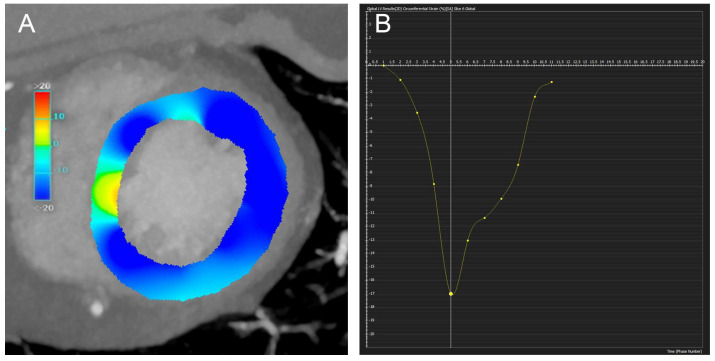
Computed tomography based strain. The circumferential strain measurement of the left ventricle (panel (A)), with the corresponding strain curve (Y−axis) over time (frame number, X−axis) (panel (B)).
